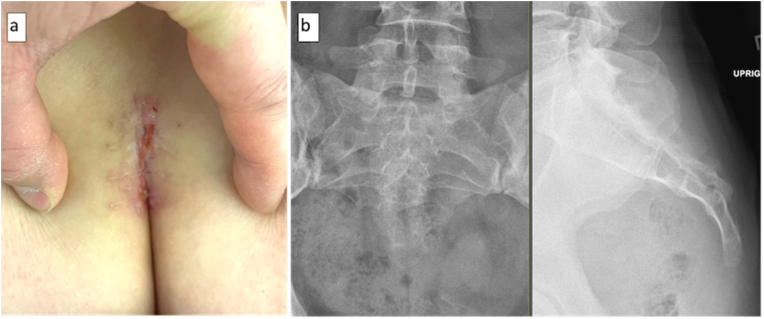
(a) Surgical site at four-week post-operative follow up. (b) Post-operative coronal and sagittal x-rays showing absence of the coccyx.
